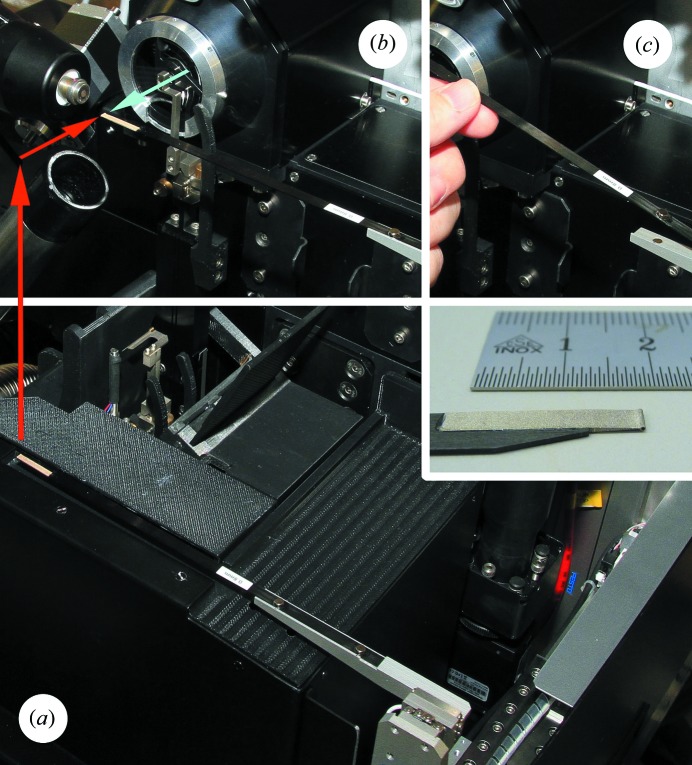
(*a*) Beamstop in the parked position, lowered (red arrow) with respect to the sample position (*b*) at the X-ray beam focus (tip of blue arrow). (*c*) Carbon blade fixation to beamstop mover *via* magnets and a reference bracket. Insert: wire-eroded Ag beamstop tip glued to a carbon blade.
